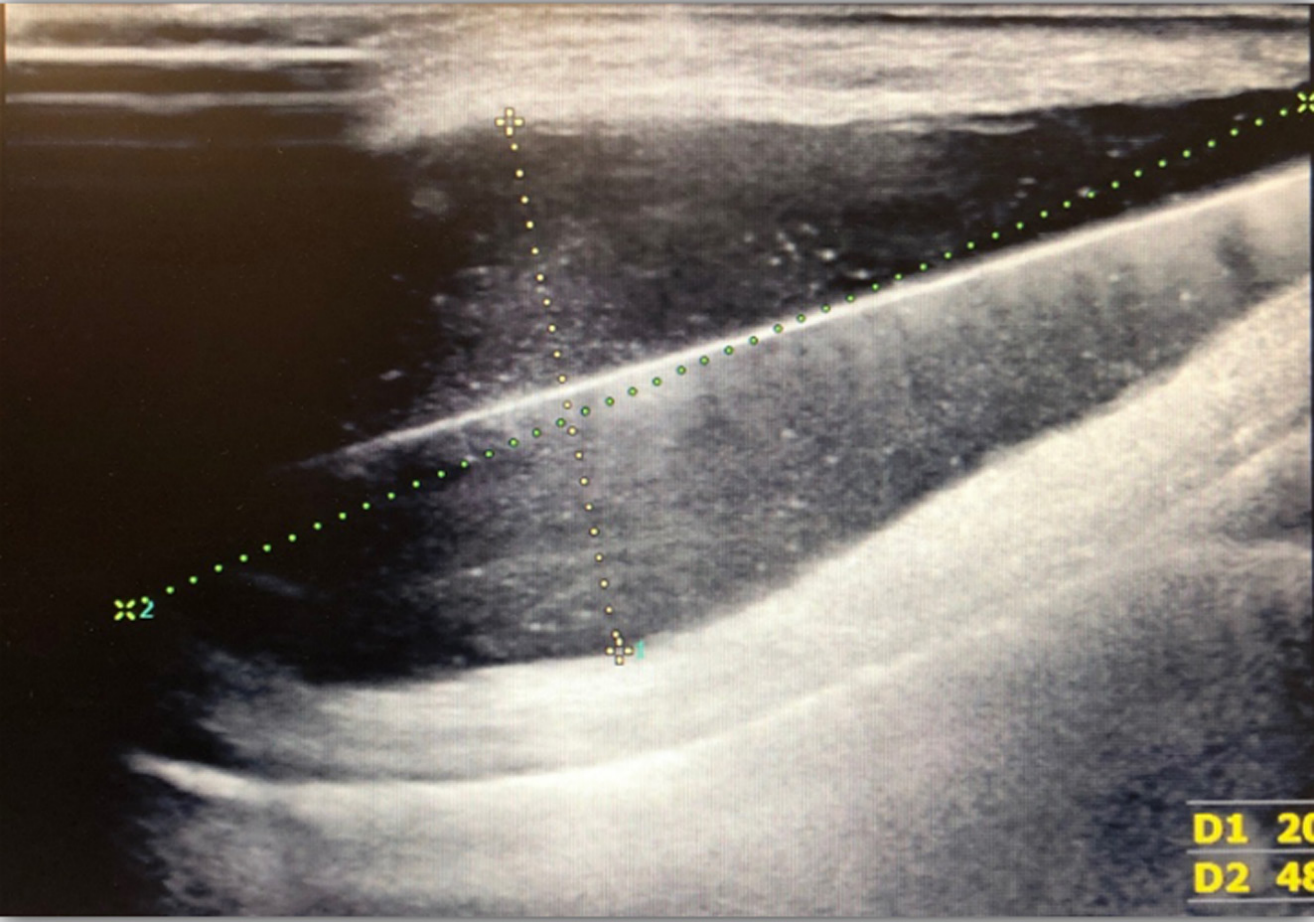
A superficial ultrasound of the tissues of the left subclavian region. It shows a 54 × 49 × 20 mm reservoir of echogenic fluid surrounding the internal pacemaker at a depth of 5 mm, the wall of the reservoir with visible vascularization.
